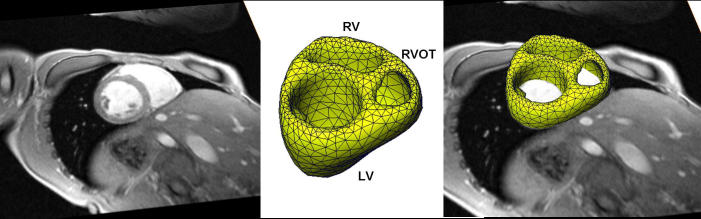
The left panel shows a MRI cardiac short axis scan (slice thickness 6mm). The middle panel shows a triangulated and remeshed surface model of individual cardiac anatomy (LV=left ventricle; RV=right ventricle; RVOT=right ventricular outflow tract). The right panel shows the fusion of MRI data and the individual cardiac model. (Figure modified from Berger T et al,  Single-beat noninvasive imaging of cardiac electrophysiology of ventricular pre-excitation. J Am Coll Cardiol 2006; 48: 2045-52. with permission. Copyright Elsevier, American College of Cardiology, 2006)
